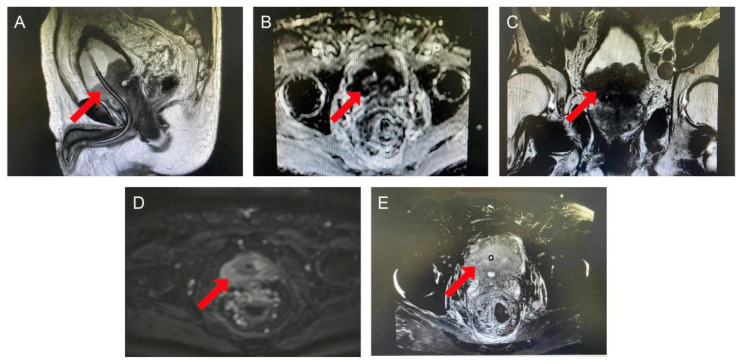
MRI images of the prostate. The figure indicates a mass in the prostate, involving the posterior wall of the bladder, bilateral seminal vesicles, and the anterior wall of the distal rectum. Lymph nodes in the pelvic wall and inguinal area were discovered to be enlarged, accompanied by numerous abnormal signal spots in the pelvis. (A–C) T2WI images of prostate cancer in sagittal, axial, and coronal views. There is a lesion in the prostate, which appears as a low signal. (D) DWI images of prostate cancer, with the tumor exhibiting a high signal. (E) ADC images of prostate cancer, indicating a lower ADC value for the tumor. The red arrow points to the prostate cancer.
